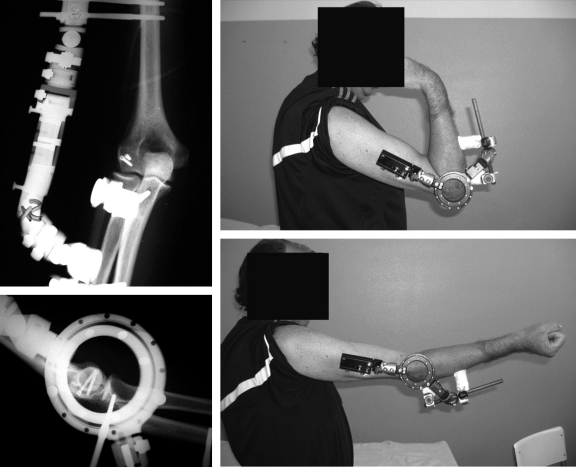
Case 4. Postoperative radiographs showing internal fixation with screws and hinged fixator. Photographs 3 weeks after surgery showing recovery of motion.
